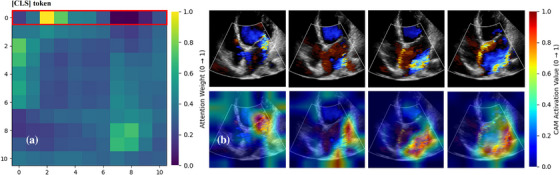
Activation maps of the attention‐based MR Detection model in Case 1 (A4C‐Color). (a) Self‐attention matrix covering 11 input frames, where index 0 corresponds to the [CLS] token. MR events occur between frames 1–6; row 0, highlighted by the red box, shows the attention weight assigned to each frame by the [CLS] token—warmer colors indicate higher attention (see color bar on the right). The model exhibits high attention to frames 1–6, consistent with clinically relevant MR periods. (b) Top row: Raw color Doppler echocardiographic frames from the MR sequence. Bottom row: Corresponding attention heatmaps superimposed on the same frames. The heatmaps show that the model primarily attends to the color Doppler information from the mitral valve orifice to the left atrium.
